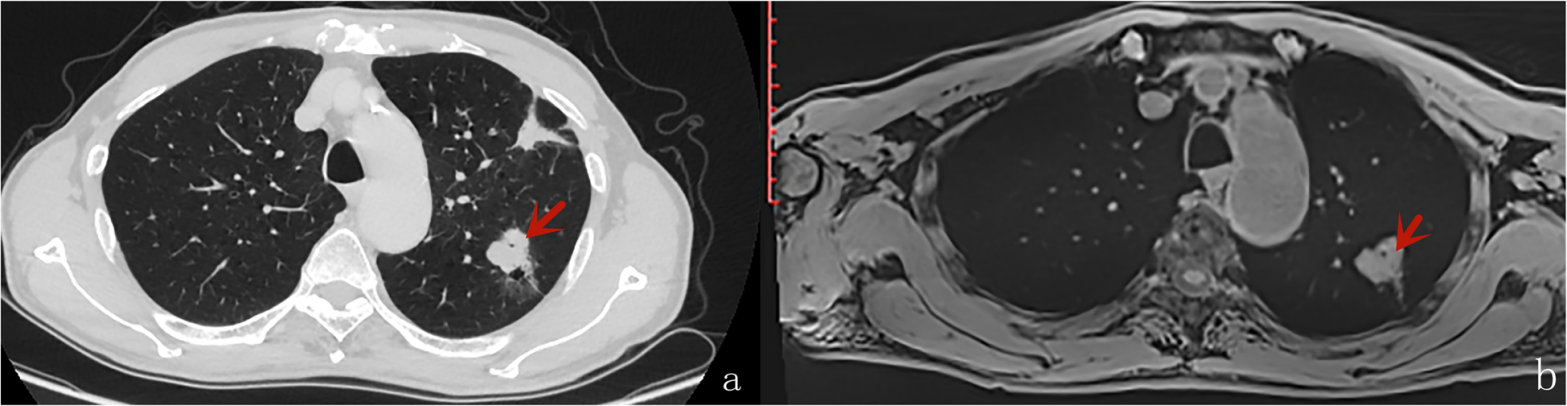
A male with small cell carcinoma, bubble-like attenuation was visible inside the lesion (the red arrow)in axial CT (A) and MR (B)
